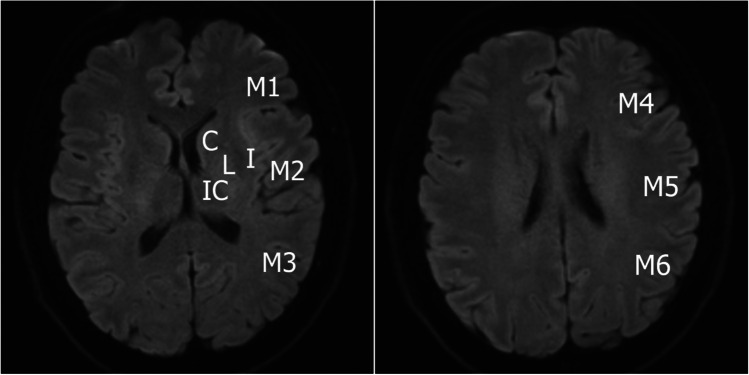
DWI image depicting ten defined regions of the middle cerebral artery territory. One point is subtracted for particular region affected by early ischaemic changes
